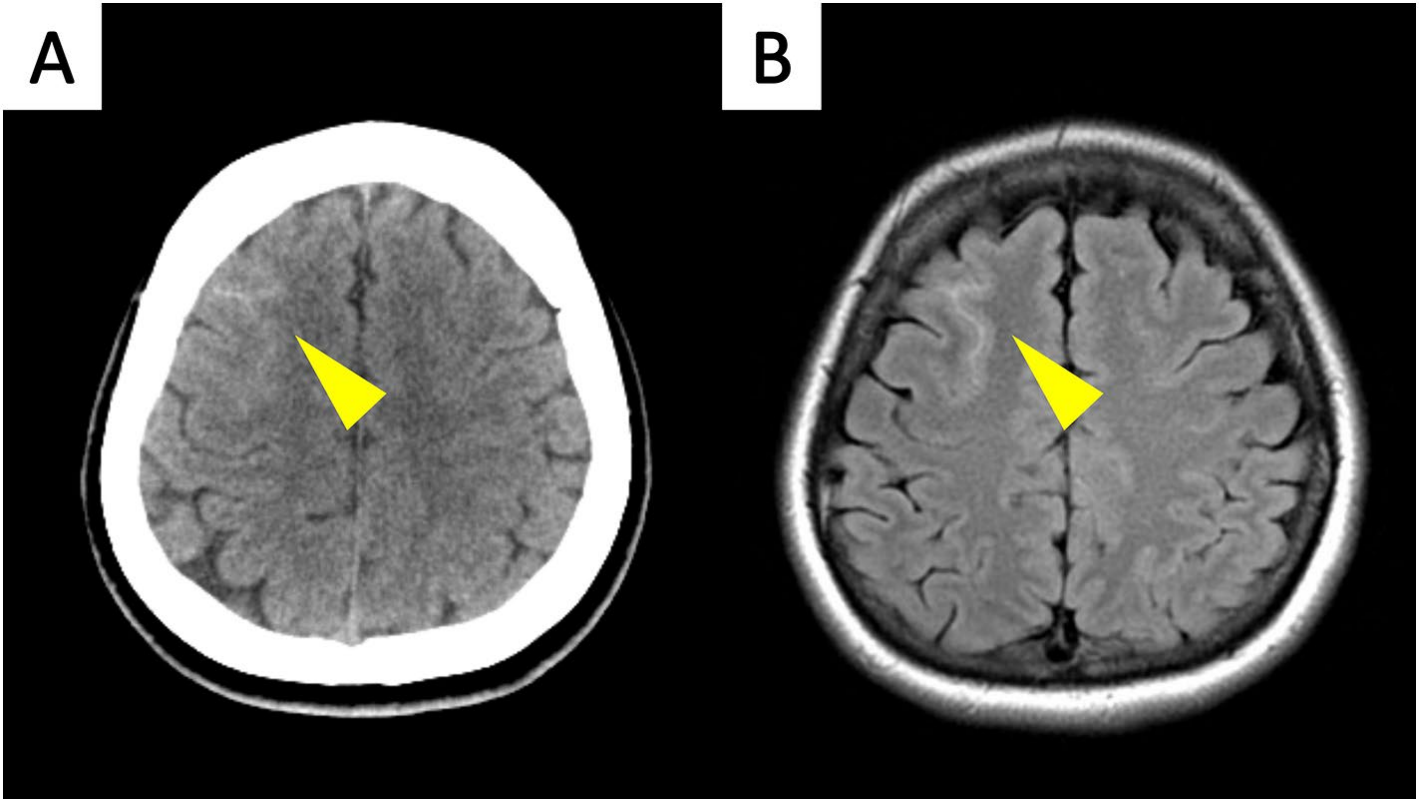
Diagnostic Imaging of Subarachnoid Hemorrhage. (A) Brain CT revealing a right parietal convexity subarachnoid hemorrhage (arrowhead). (B) T2-weighted FLAIR MR image obtained on admission showing high signal intensity in the right parietal convexity (arrowhead)
